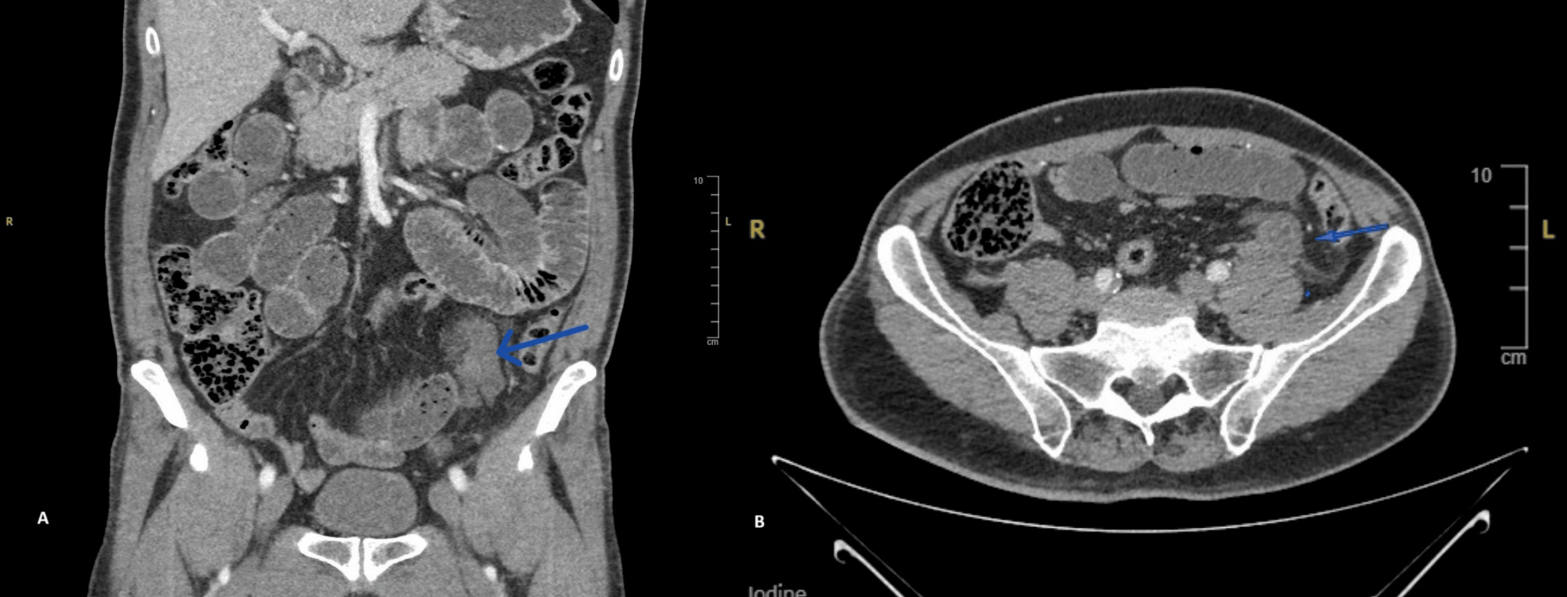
Computed tomography (CT) scans. (A) Coronal view: The image shows the overall abdominal anatomy with multiple dilated small bowel loops, suggestive of obstruction. (B) Axial view – low pelvis: The image demonstrates clustered small bowel loops and a transition point in the left iliac fossa (indicated by the blue arrow), adjacent to the sigmoid colon. This corresponds to the location of the intersigmoid internal hernia.
